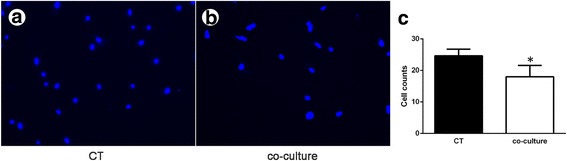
ADSC-CM depresses the invasive abilities of keloid fibroblasts. a, b As determined by transwell® assay, the migratory keloid fibroblasts were visualized by imaging the nuclei labeled with DAPI. c The number of migrated cells was counted in three randomly selected fields. P* < 0.05, versus control (CT)
